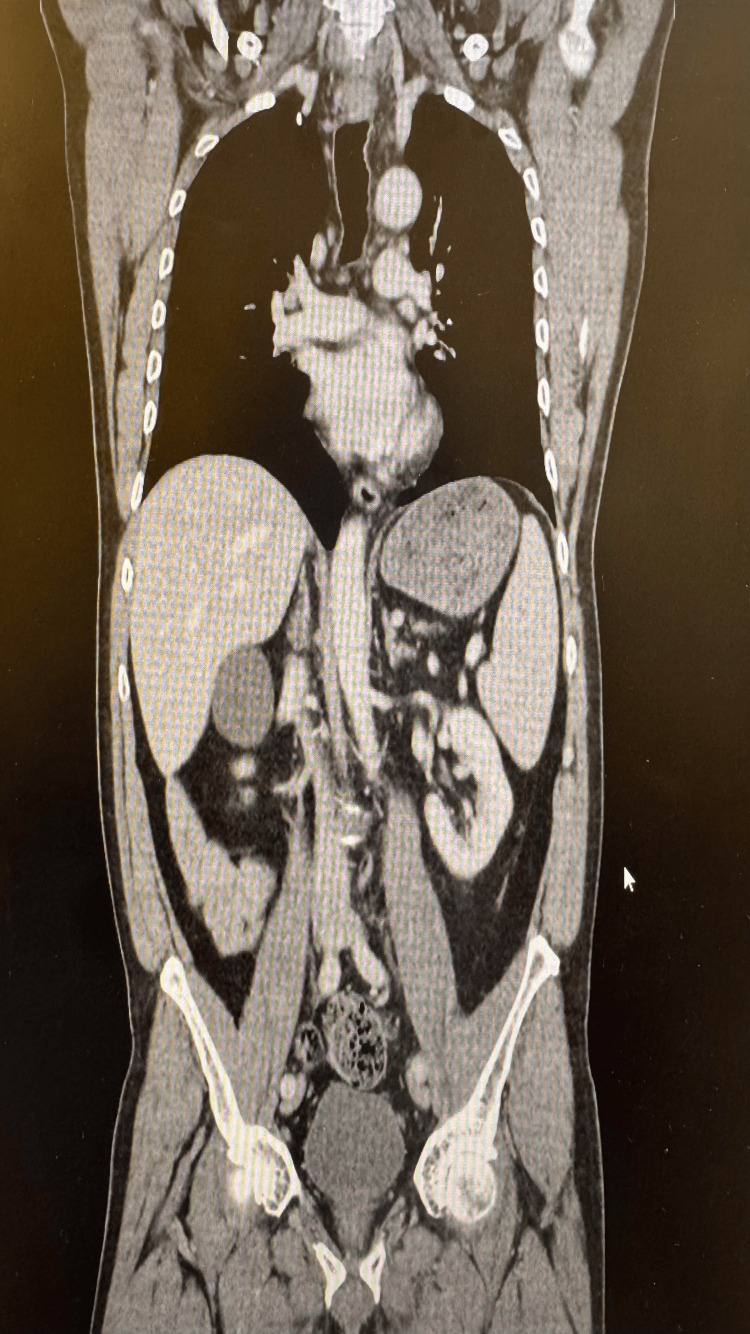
Apical view of CT imaging showing no acute abnormalities.
